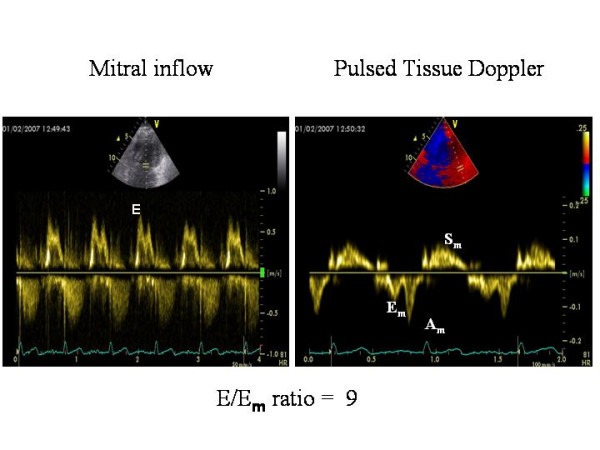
Standard Doppler derived transmitral inflow pattern (left panel) and Pulsed Tissue Doppler of the lateral mitral annulus in a HT recipient. The ratio is = .9.
